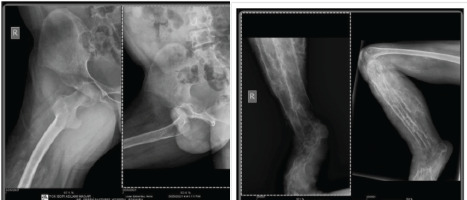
X-Ray (A) Right hip showing joint space reduction with patchy calcification (B) Knee and ankle showing osteopenia, soft tissue patchy calcification and distorted ankle joint.
